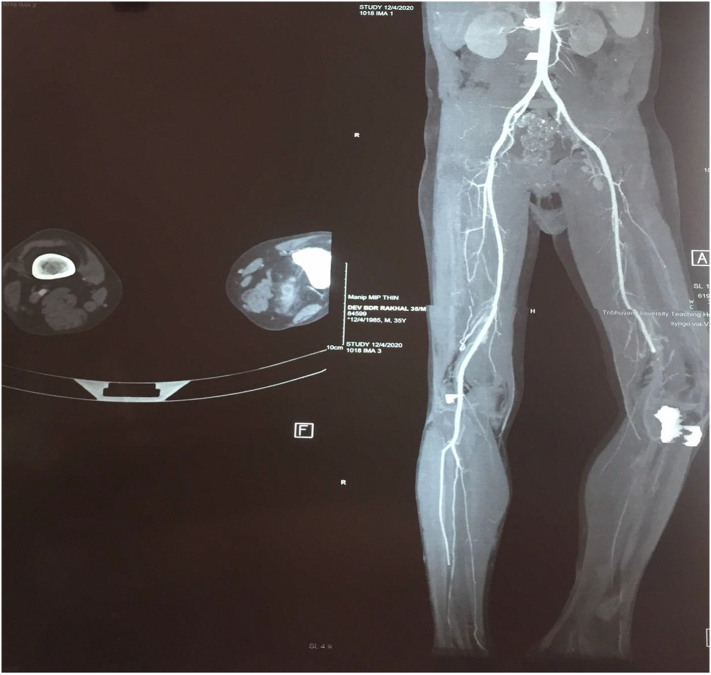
CT angiography in the left leg showing contrast opacification of the distal superficial femoral artery with abrupt cutoff at the origin of popliteal artery. Distally faint reformation of the anterior and posterior tibial artery present.
